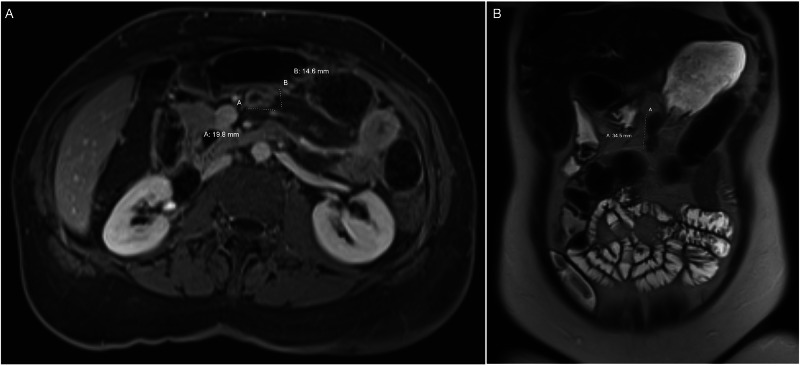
MRI enterography with and without gadolinium (A) Axial single slice and (B) coronal single slice showing a 2.0 x 1.5 x 3.5 cm transverse by anteroposterior by craniocaudal dimension ovoid enhancing lesion within the mesentery of the upper abdomen just posterior inferior to the gastric antrum.
